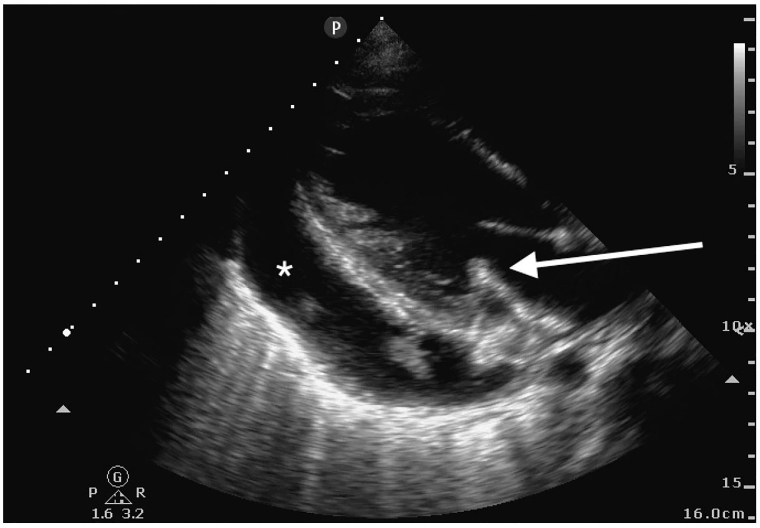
Parasternal long-axis transthoracic view showing pericardial effusion (star) and thickened posterior mitral valve leaflet (arrow).
